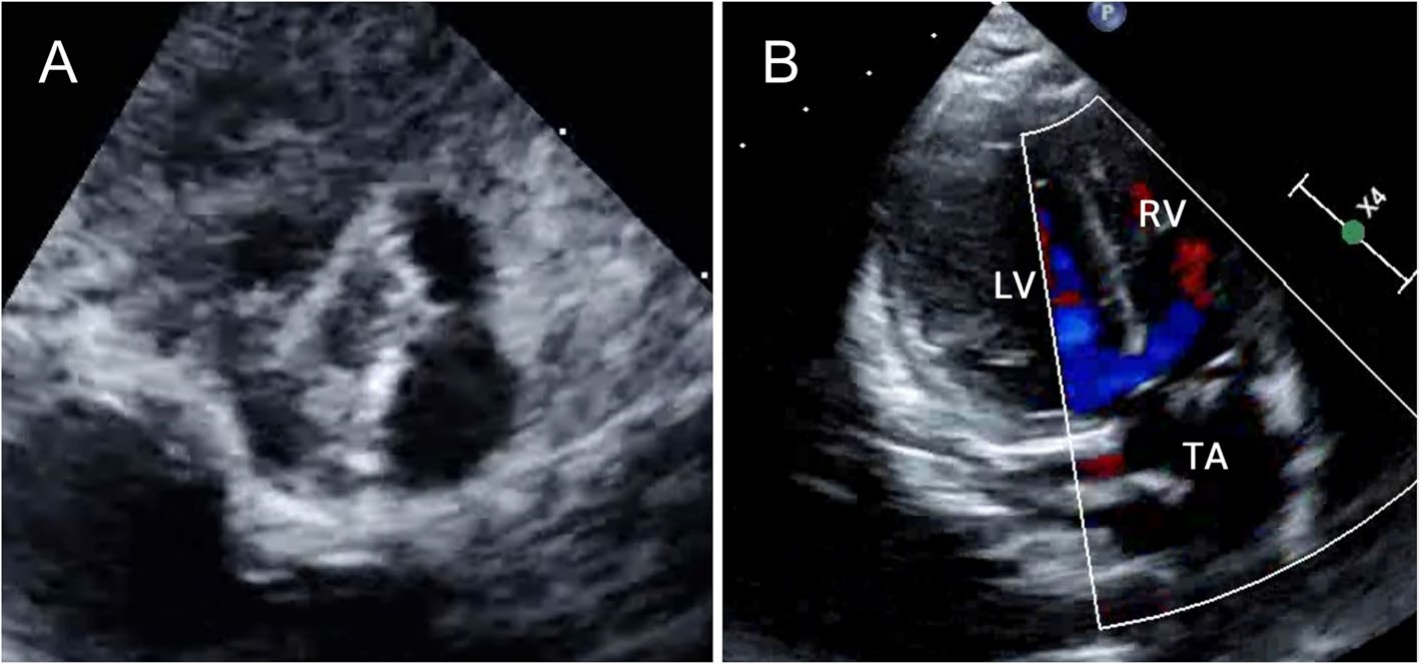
A The reconstructed truncal valve in the parasternal short-axis view. B Postoperative color-Doppler echocardiography shows complete elimination of the regurgitant jet of the truncal valve. RV, right ventricle; LV, left ventricle; TA, truncus arteriosus
